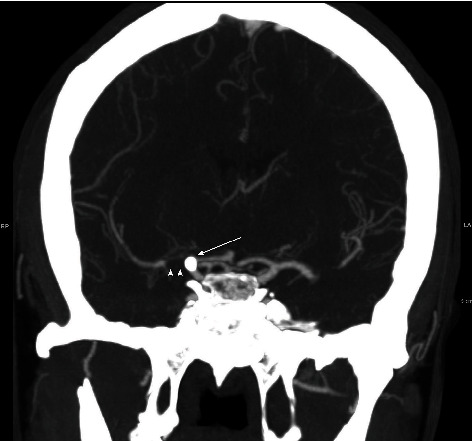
CT angiography of the head. Coronal maximum intensity projection (MIP) demonstrates occlusion of the right carotid terminus (arrow) due to calcified cerebral embolus and absent flow within the right M1 middle cerebral artery (arrowheads).
